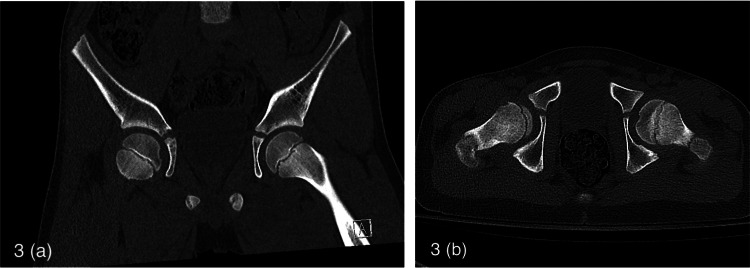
Coronal (a) and axial (b) slices of post-reduction CT scan demonstrating the position of the left femoral head in the acetabulum. No fractures or incarcerated osteochondral fragments were seen.
